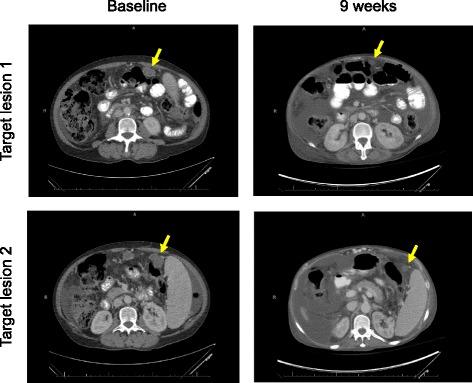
Baseline images (left) compared with 9 week CT scan (right) showing reduction in target lesion size with anti-PD-1 therapy
